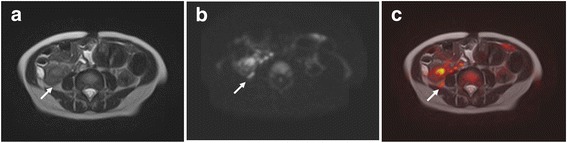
MRI findings. a T2-weighted image. b DWI. (c) T2-weighted image and DWI fusion image using medical image viewer software (EV Insite R, PSP Co., Tokyo, Japan). *Arrows*: intra-abdominal testis located below right kidney
